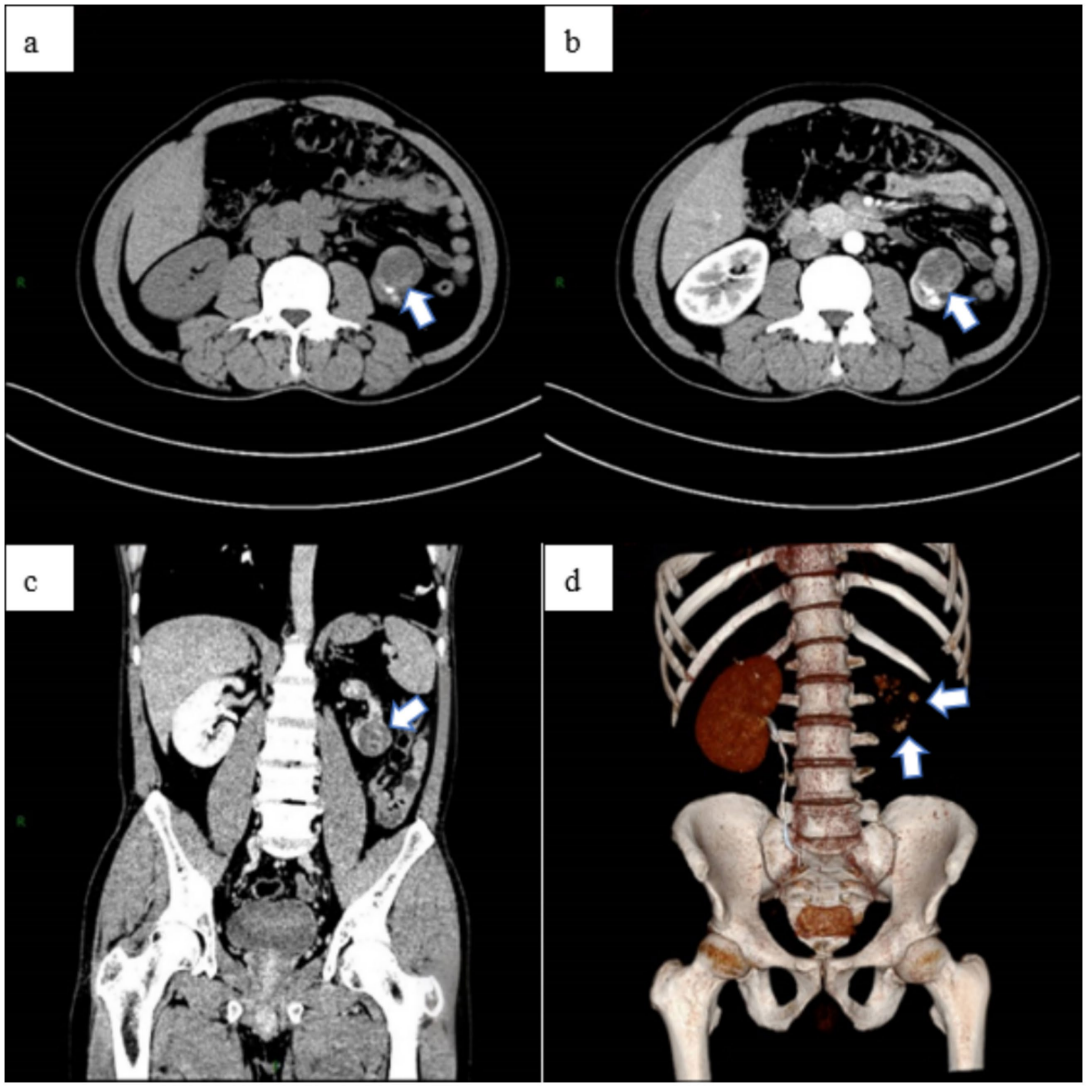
The abdominal CT scan revealed a space-occupying lesion in the left kidney, measuring approximately 28 × 29 × 31 mm (a), along with multiple stones. The abdominal enhanced CT showed uneven enhancement of the lesion (b), located in the lower pole of the left kidney (c), and a reduction in the volume of the left kidney. Additionally, the left kidney and ureter were not visualized, indicating non-functionality of the left kidney (d).
